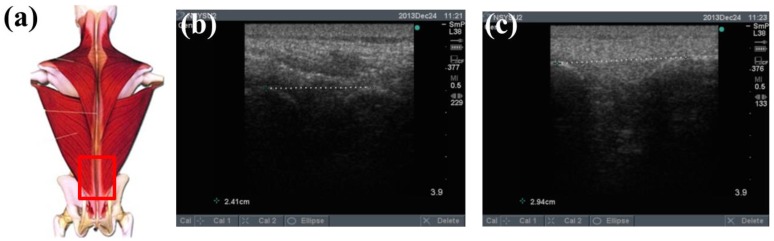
Results from ultrasound images: (a) measured lower erector spinae position; (b) preparatory action; (c) anteflexion action.
